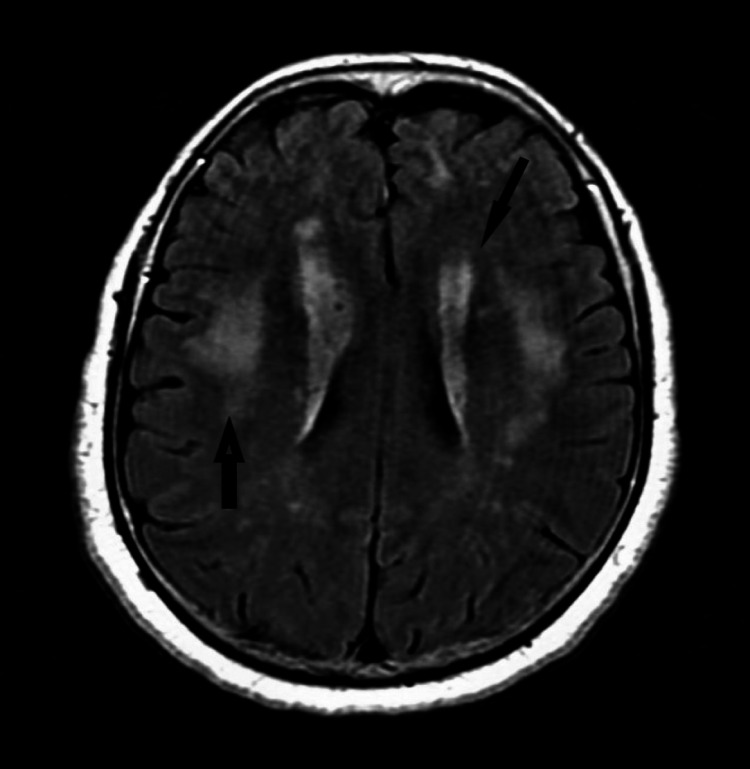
MRI Brain showing patchy areas of asymmetric subcortical lesion on FLAIR sequence images (black arrows)
